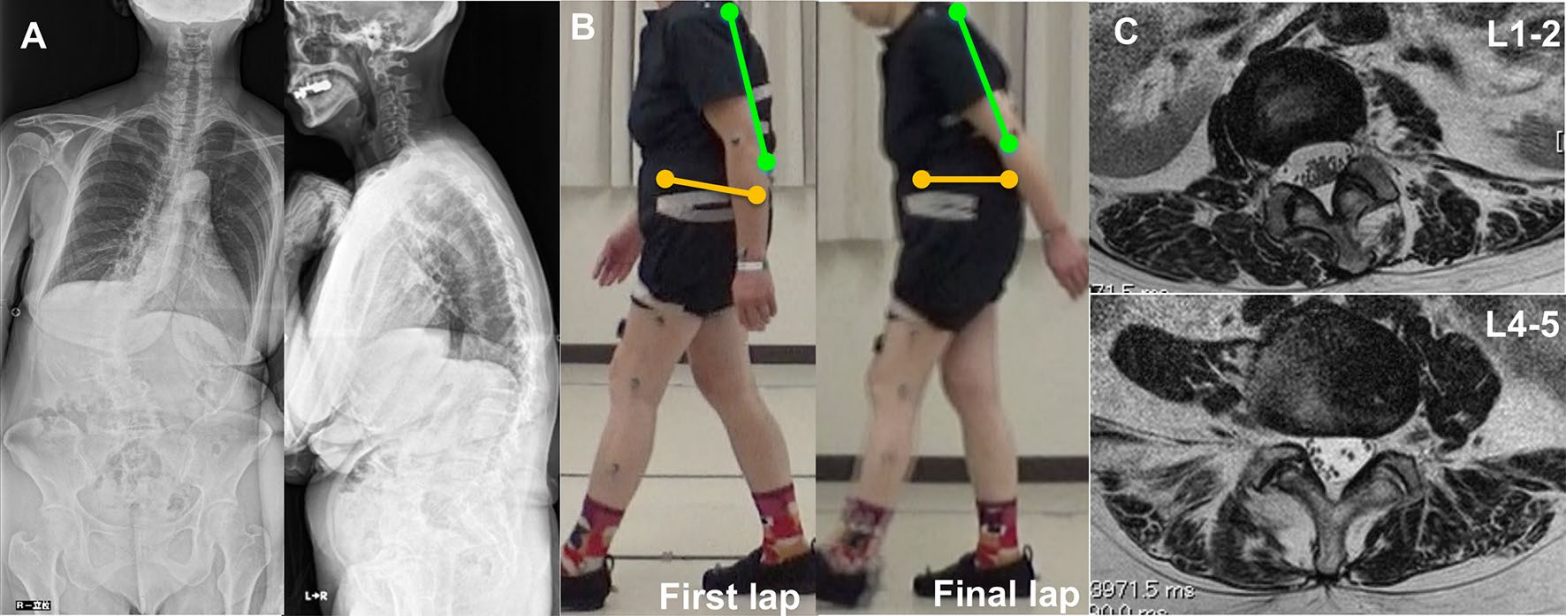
A representative case of a 63-year-old female patient with almost normal TK and increased PT, large fat infiltration rate of the lumbar erector spinae muscles and an increase of spinal kyphosis and pelvic anteversion. (A) Full-spine radiographs. SVA: 89 mm, TK: 40°, LL5.8°, PT 35°, PI: 39° (B) Posture during the three-dimensional gait motion analysis on the first and final laps. ΔSpA: 2.5°, ΔPA: 3.1° (C) T2 weighted axial MRI images at L1-2 and L4-5. The fat infiltration rate of the lumbar erector spinae muscles (L1-2: 15% and L4-5: 24%).
